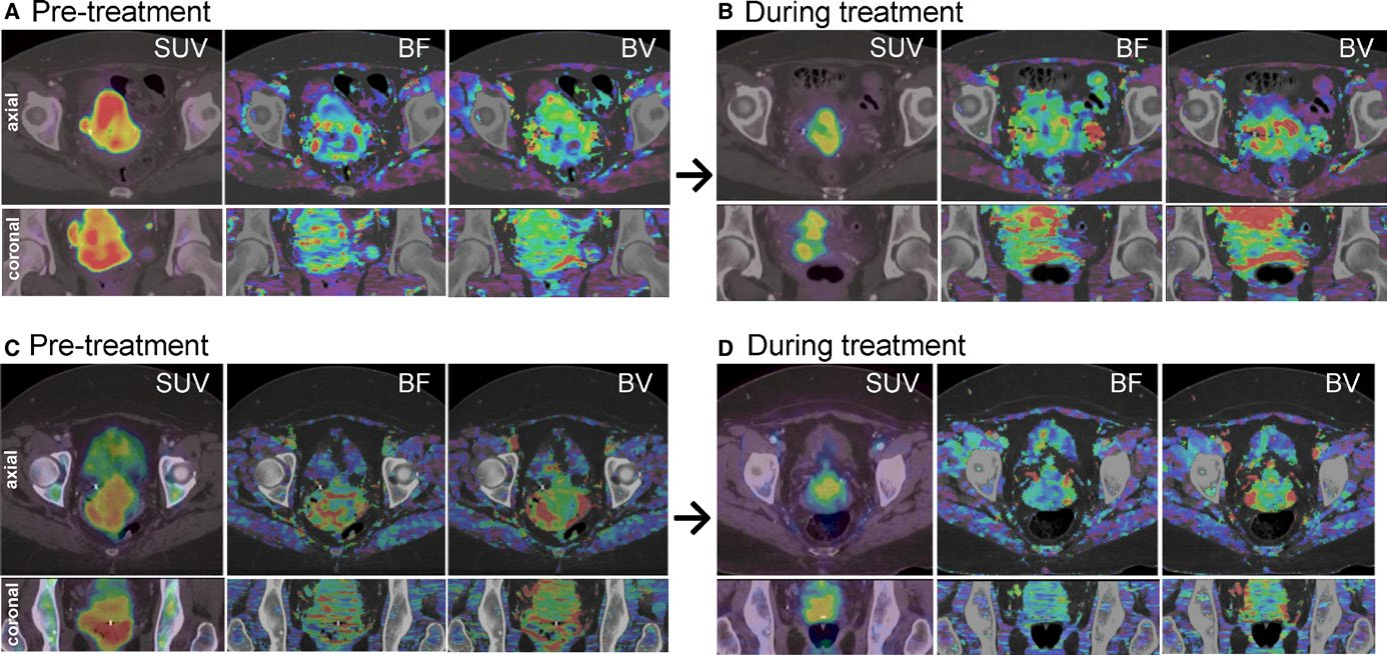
Axial and coronal slices from pre‐ and during‐treatment volumetric maps of standardized uptake value (SUV), blood flow (BF), and blood volume (BV) for 2 patients. The patient shown in (A) and (B) exhibited complete metabolic response at initial posttreatment follow‐up, while the patient shown in (C) and (D) exhibited new distant disease at their same follow‐up
